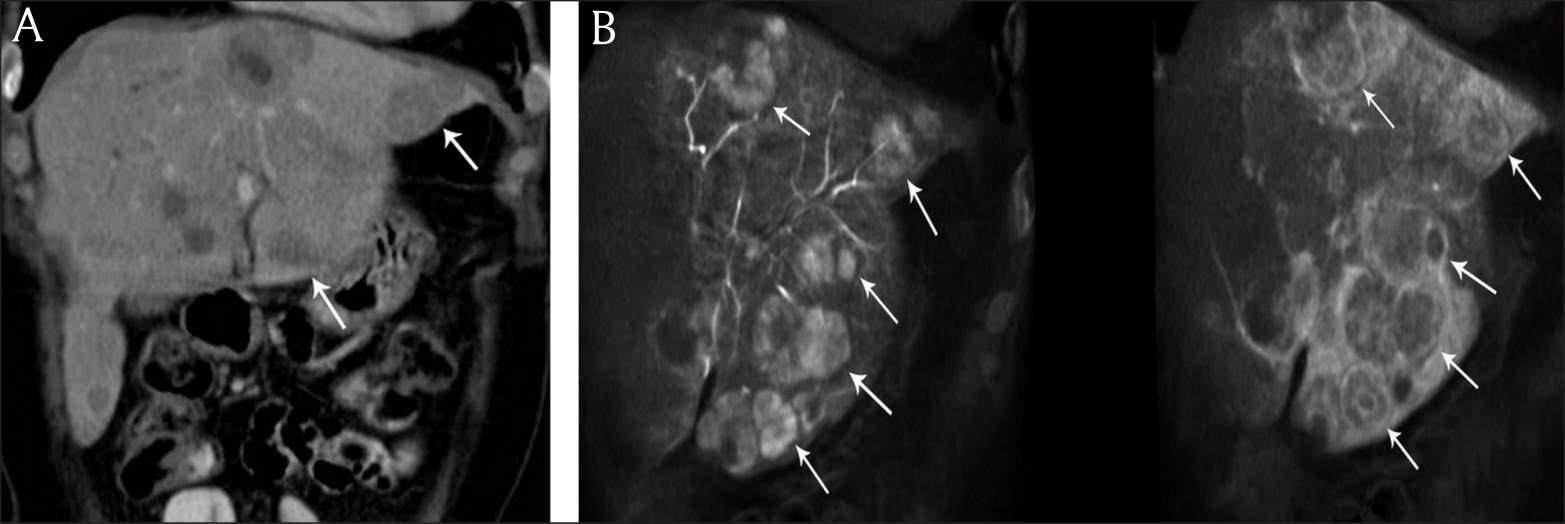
A) Coronal reconstructed contrast-enhanced computed tomography and B) cone beam computed tomography imaging in an 58-year-old woman presenting with bilobar neuroendocrine liver metastases. Increased number of liver metastases (white arrows) are identified on cone beam computed tomography imaging versus contrast-enhanced computed tomography.
